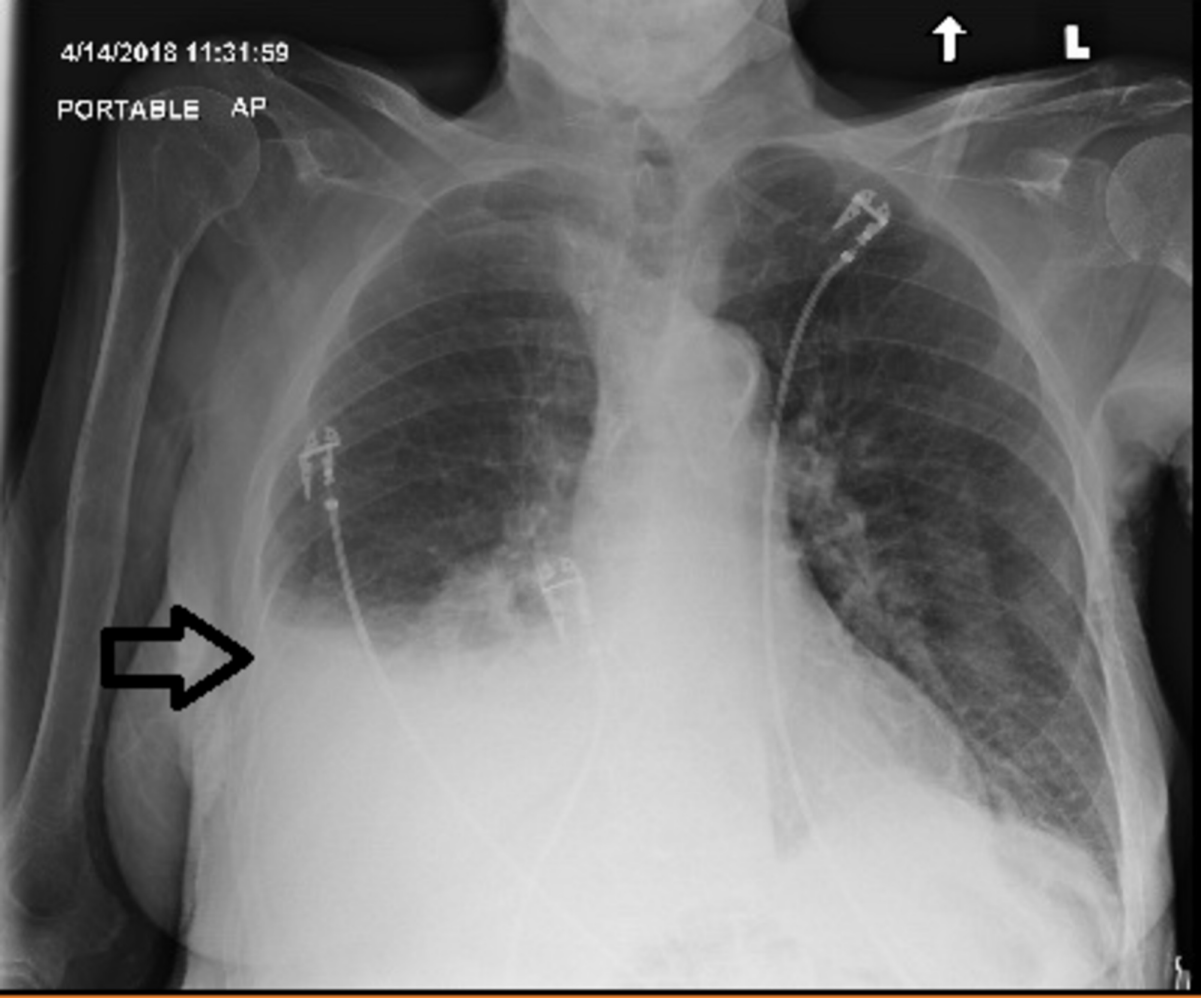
Radiograph of chest anterior-posterior view; arrow indicates the postoperative pleural fluid collection expected after surgical correction of the diaphragmatic hernia
